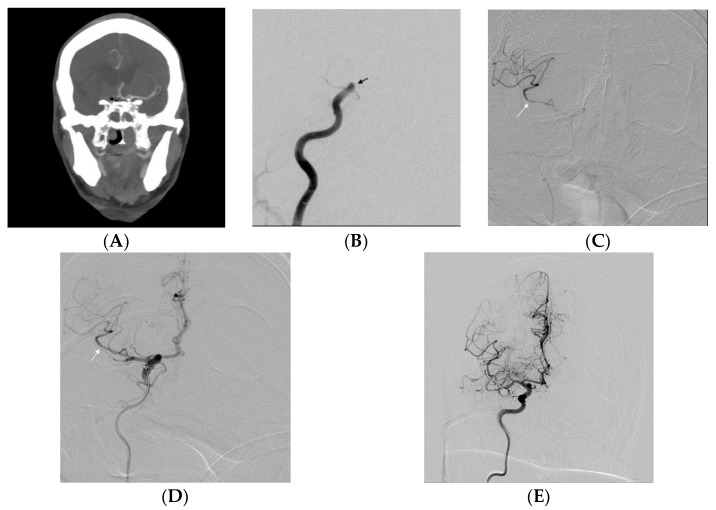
(A) Preprocedure CTA of the head (coronal image) indicated that the terminal end of the right ICA was occluded (black arrow). (B) DSA of the right common carotid artery revealed that distal ICA was occluded (black arrow), which includes the supraclinoid segment of the ICA. (C) After the occlusion site was passed with a microcatheter (white arrow), a gentle contrast injection was performed through the microcatheter to verify the placement beyond the clot. (D) A Solitaire 6 × 40 mm stent (white arrow) was used, and 6F intermediate catheter (Sofia) was advanced closer to the thrombus for local aspiration after the stent retriever removal. (E) TICI 2B recanalization was observed after a 1-pass.
